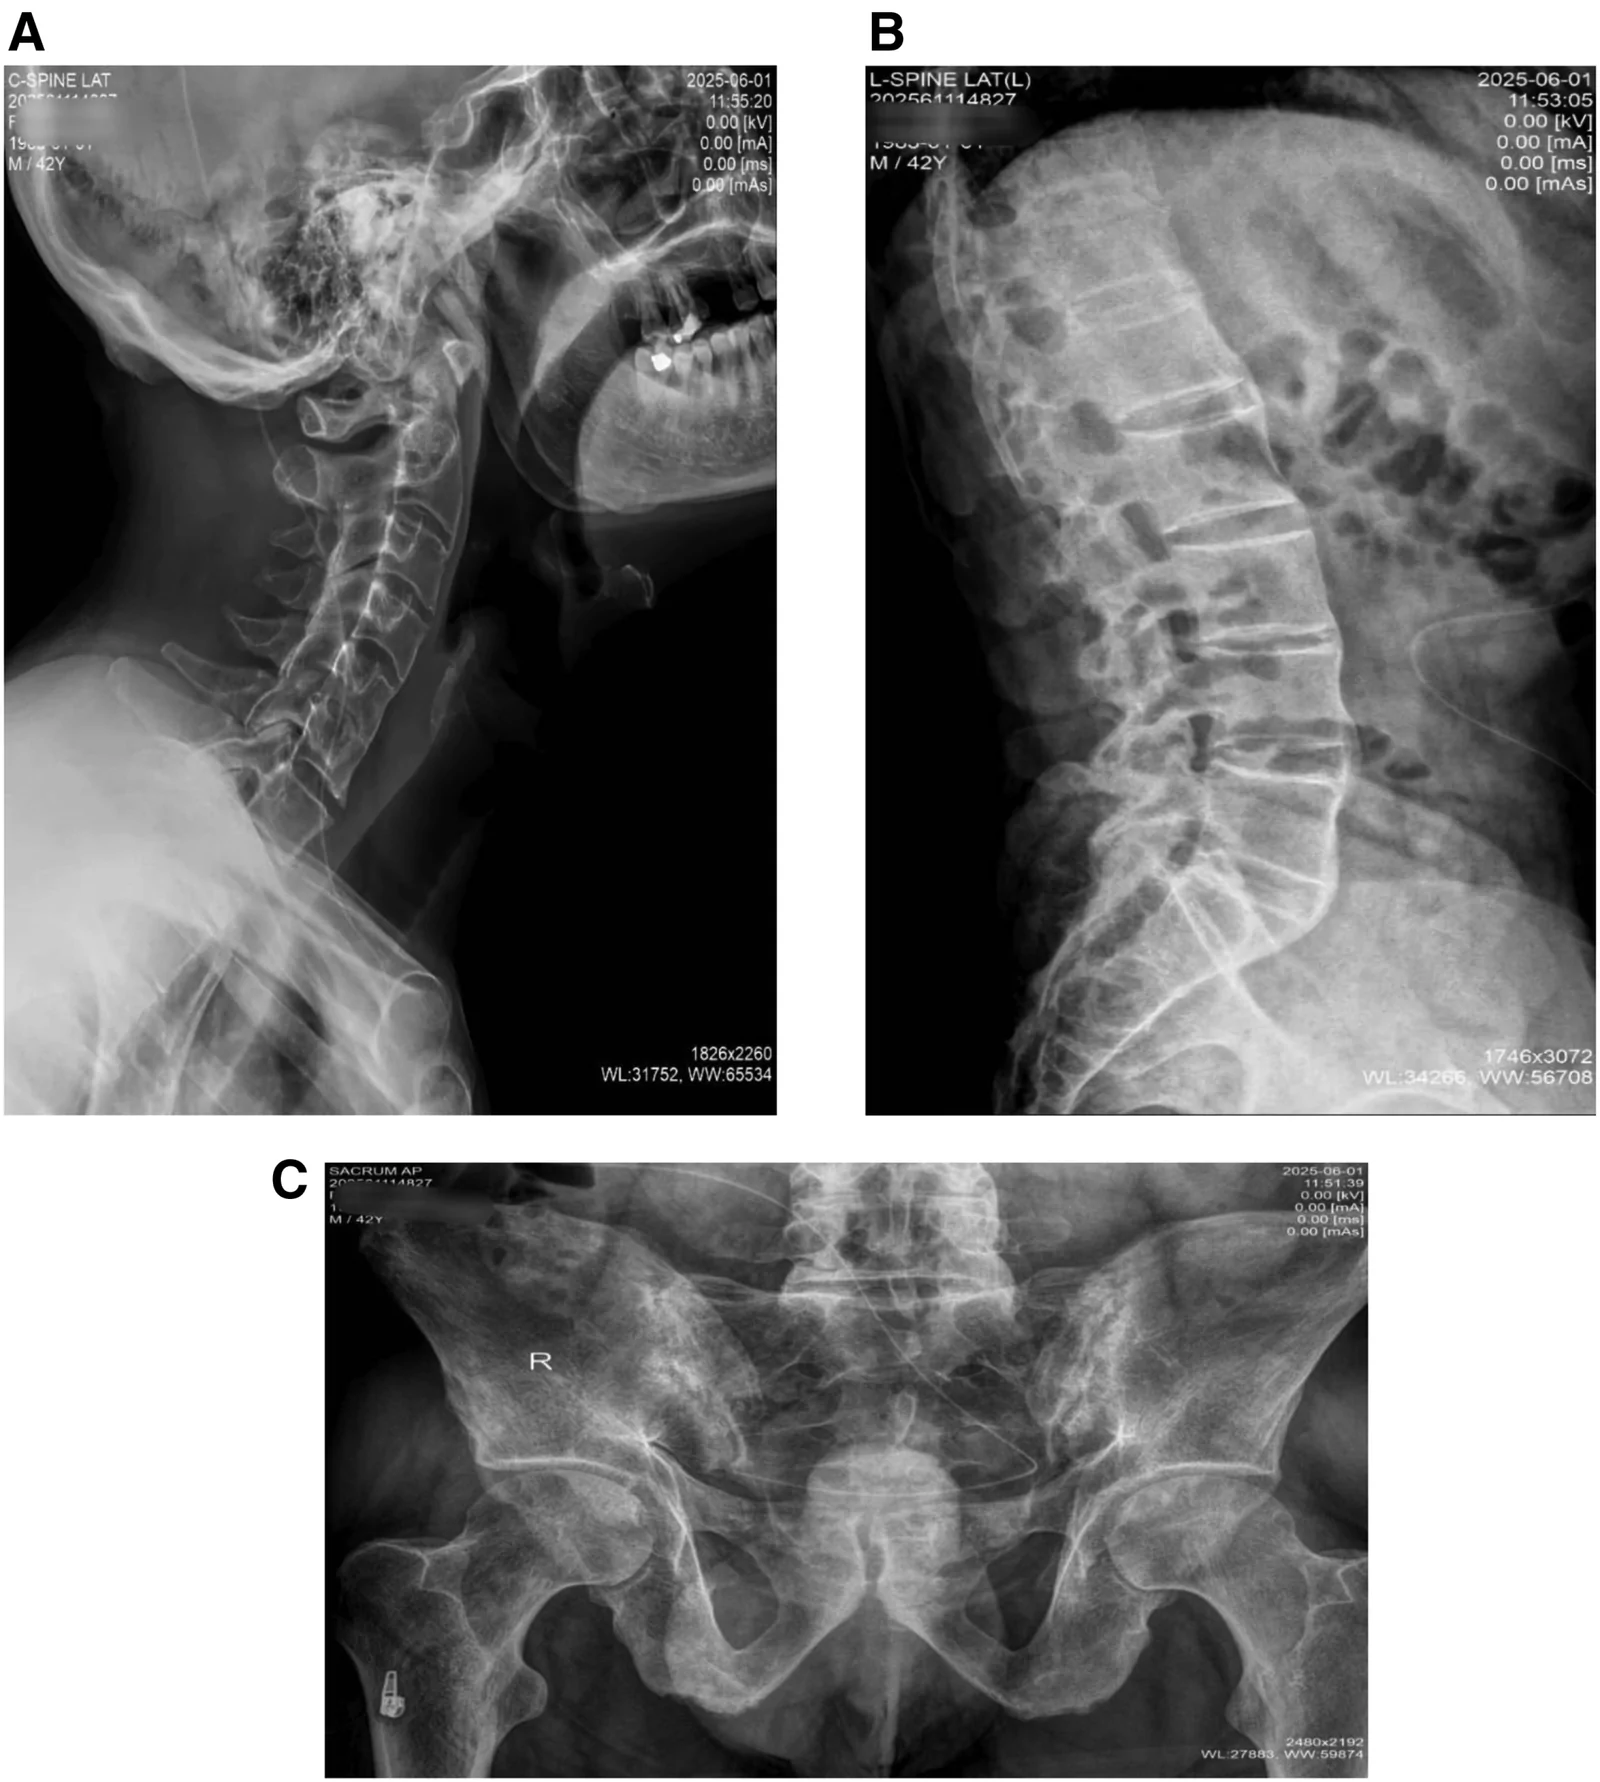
(A) C-Spine LAT Cervical spine radiograph demonstrates anterior ossification and early ‘bamboo spine appearance. (B) L-Spine LAT Lateral lumbar spine shows squaring of vertebral bodies and early syndesmophyte formation. (C) Sacrum AP: Bilateral sacroiliac joint erosions, sclerosis, and early intra-articular fusion.
